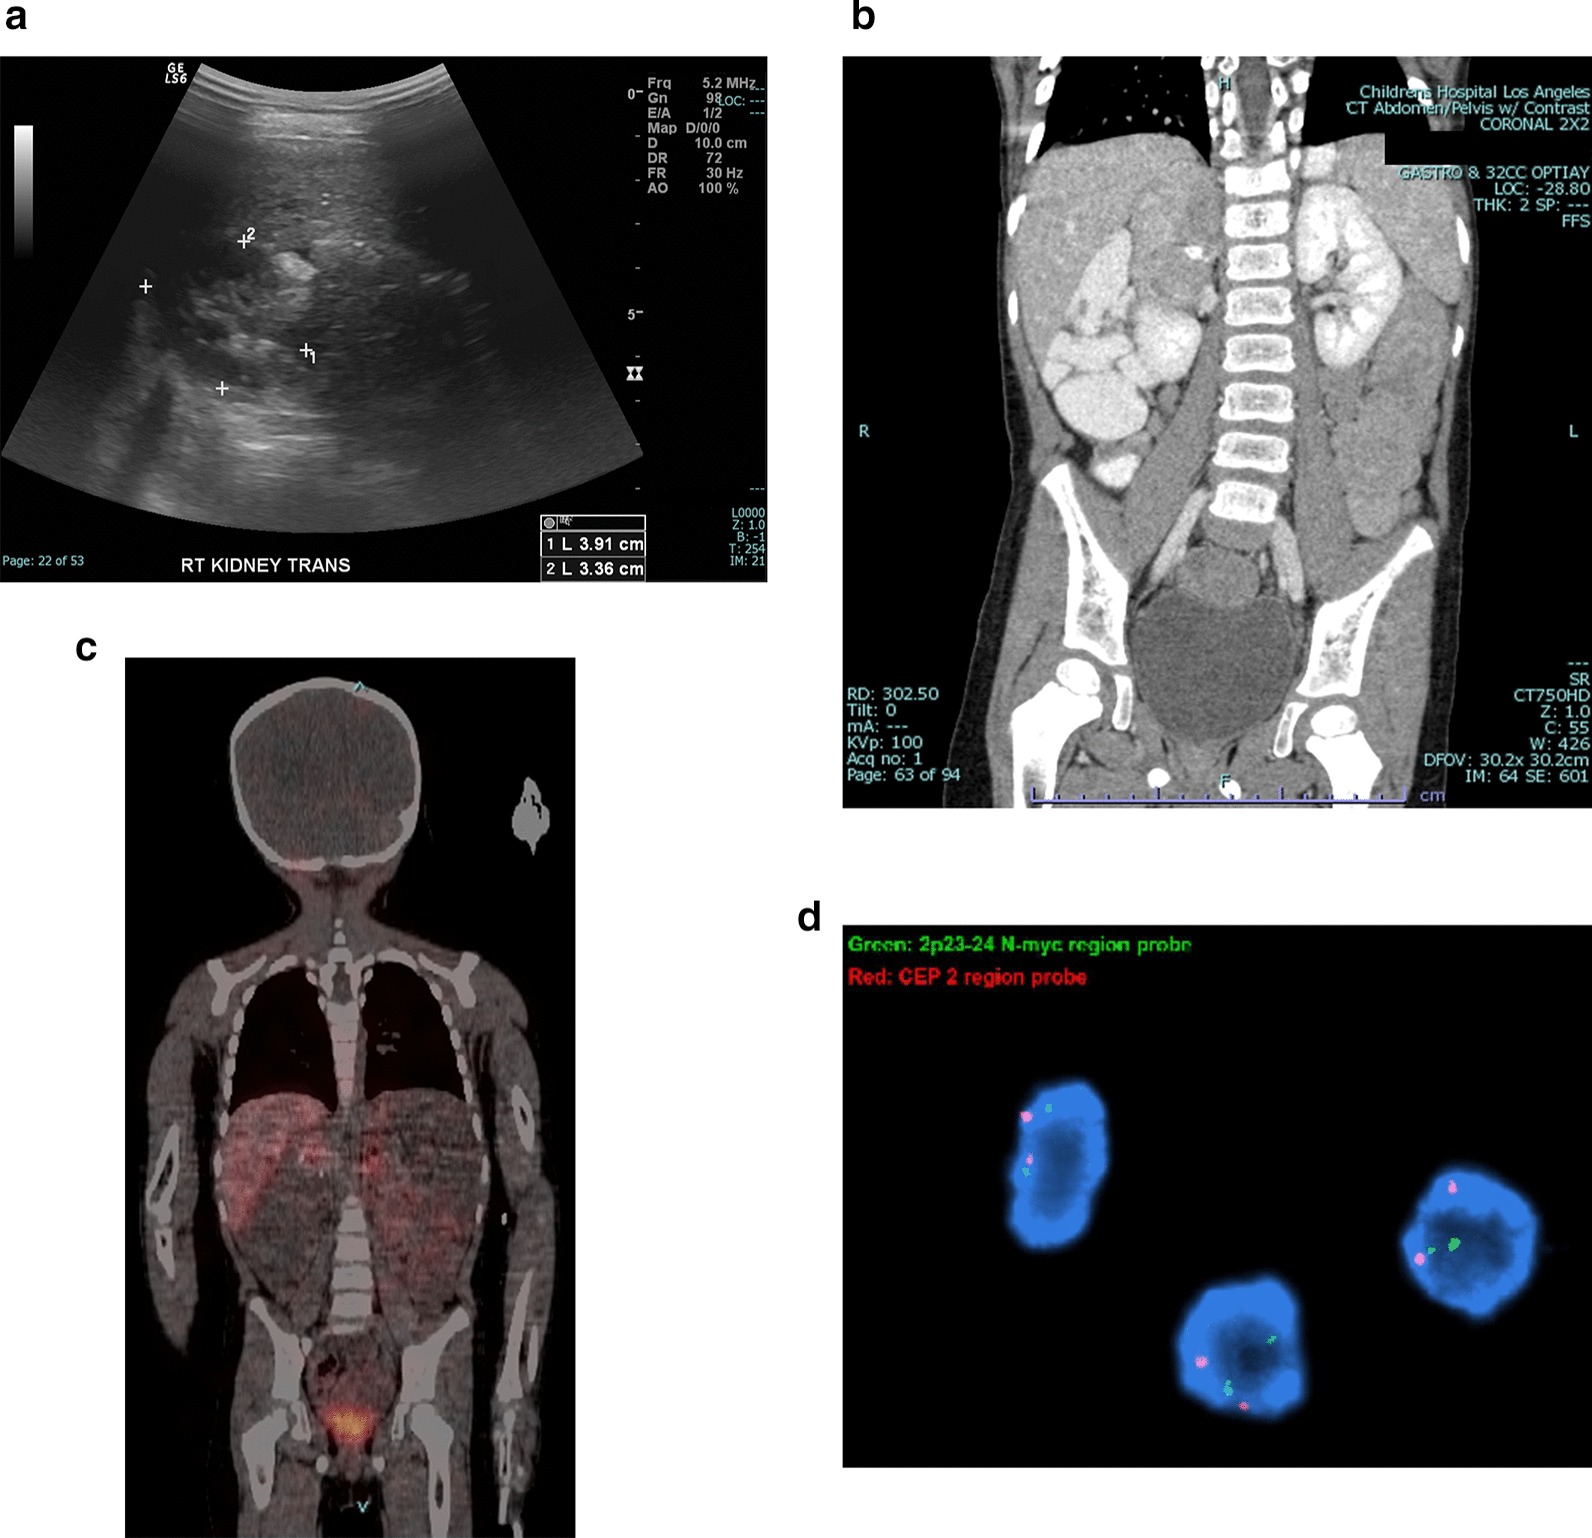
a Renal ultrasound for *Case 3* showing a heterogeneous complex mass in the area of the right kidney. b CT scan of abdomen and pelvis showing a 6.4 cm × 4.2 cm × 3.4 cm heterogeneous calcified mass replacing the right adrenal gland concerning for neuroblastoma. No regional or distant lymphadenopathy was noted. c MIBG study showing activity in the right adrenal gland without evidence of avid metastatic disease. d FISH of the final tumor did not demonstrate *MYCN* amplification
